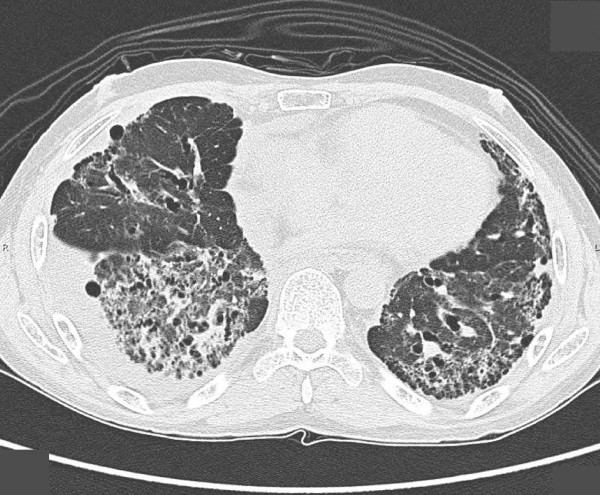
HRCT chest scan reveals thickening of interlobular septa with sub-pleural emphysema and tubular bronchiectasis of right lower lung.
